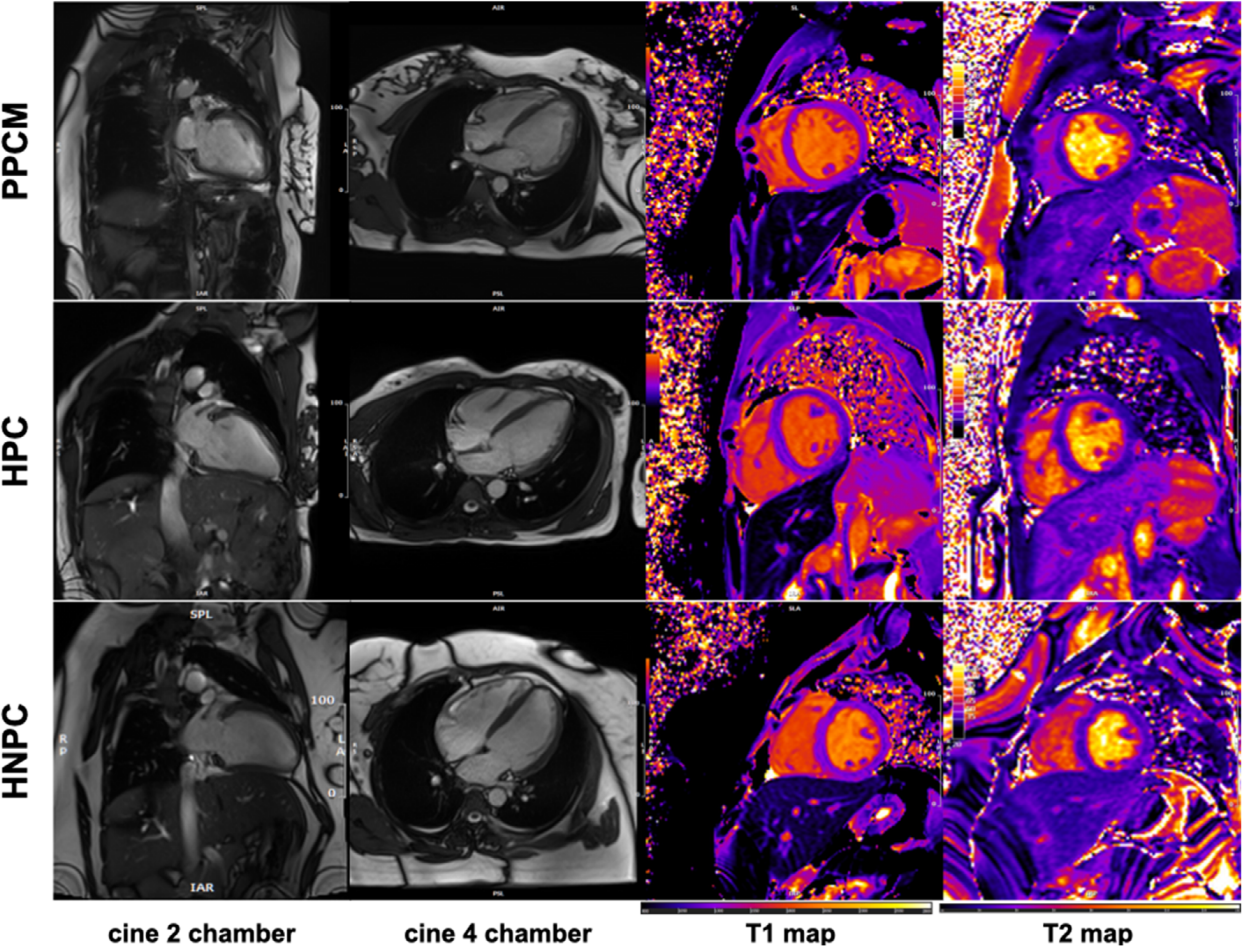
Representative cardiovascular magnetic resonance images of peripartum cardiomyopathy (PPCM) patients, healthy postpartum controls (HPC) and healthy non‐postpartum controls (HNPC) displaying a cine two chamber (first row), cine four chamber (second row) as well as short axes at papillary muscle level of native T1 map (third row) and T2 map (fourth row).
